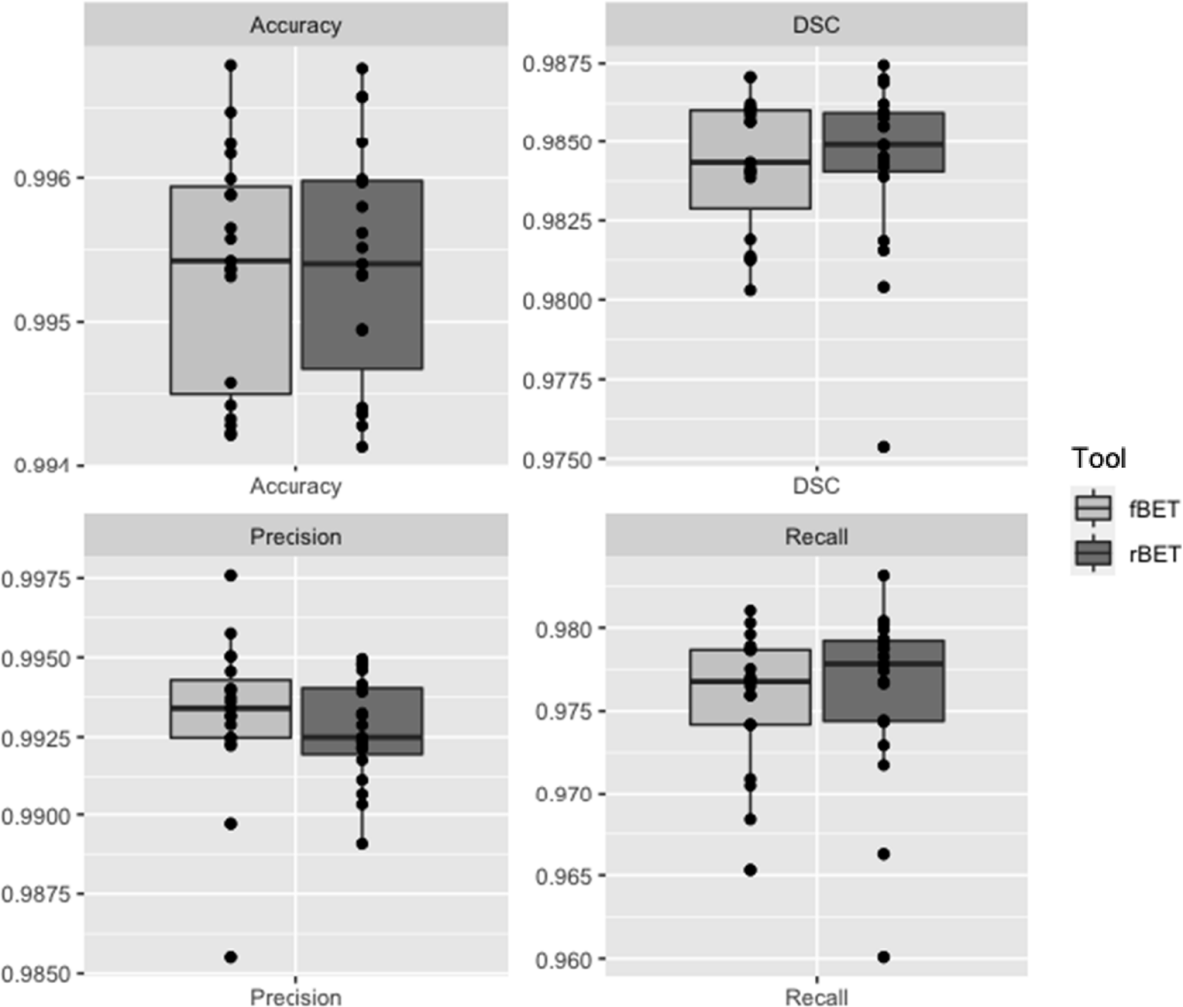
Comparison of performance metrics using CT brain extraction tools in 20 patients. *DSC = Dice similarity coefficient, fBET = FSL adapted tool, rBET = CNN-based tool*
